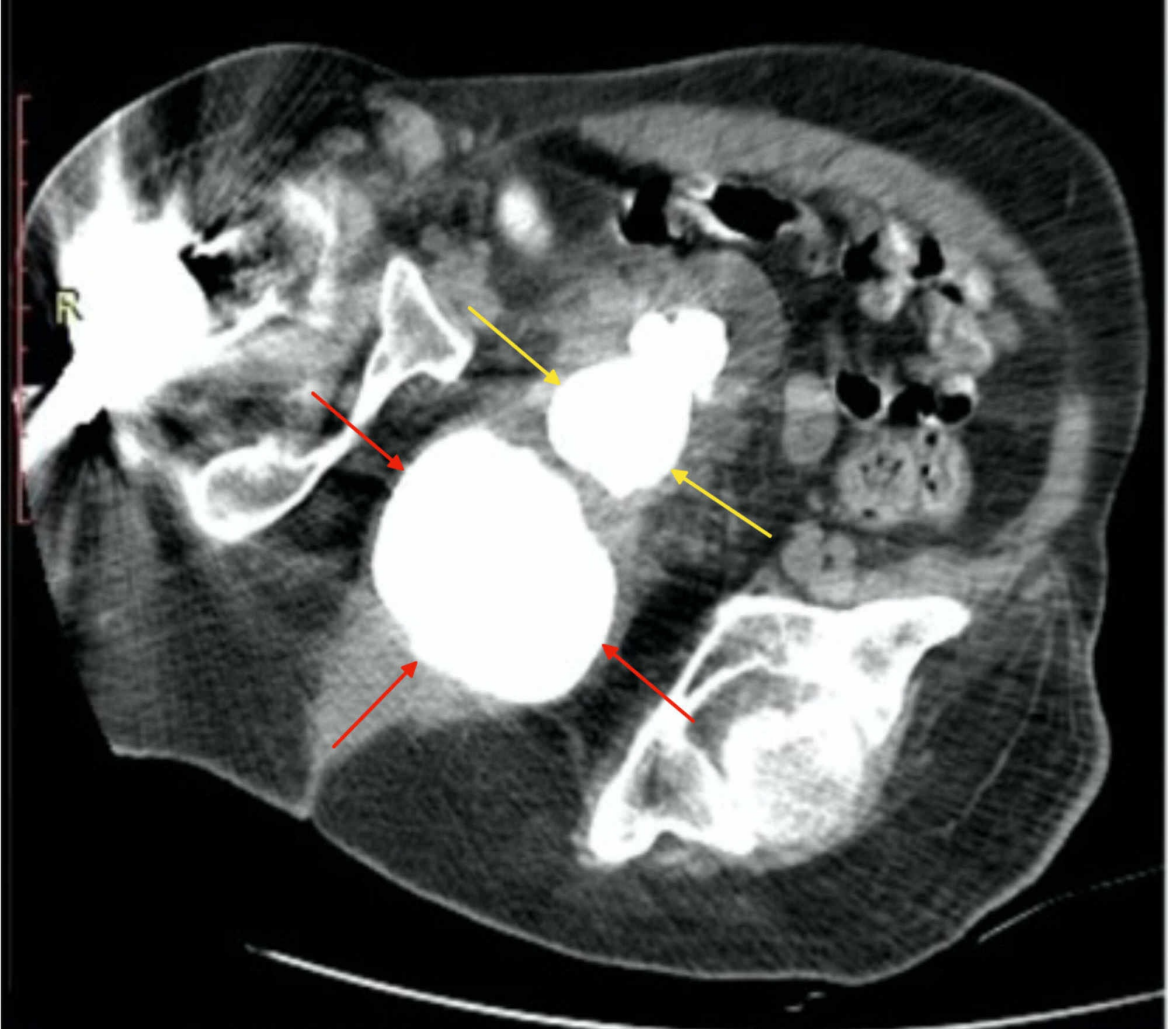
Pelvic CT reveals an osseous transformation of the prostate (red arrows) and multiple bladder stones (yellow arrows)
